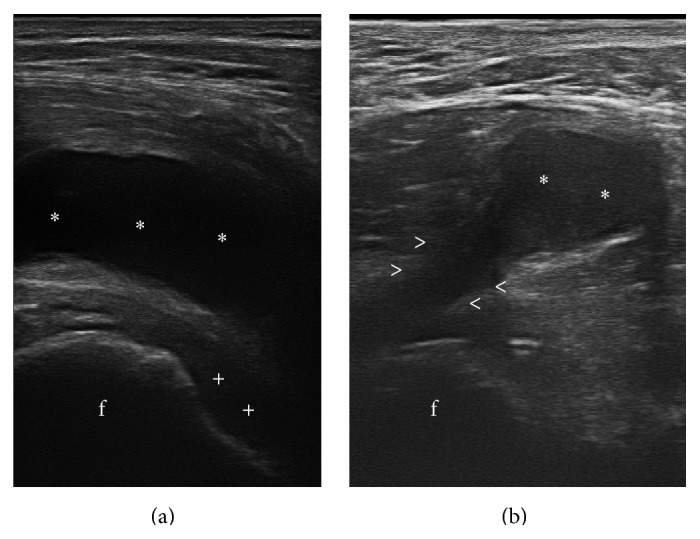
Ultrasound examination of the hip. (a) Longitudinal scan revealing the presence of a neoformation with fluid content (asterisks) anterior to the right hip. In the hip joint, an effusion (+) is present. (b) Semicoronal scan showing the connection between the joint and the formation (arrowheads), f: femoral head.
